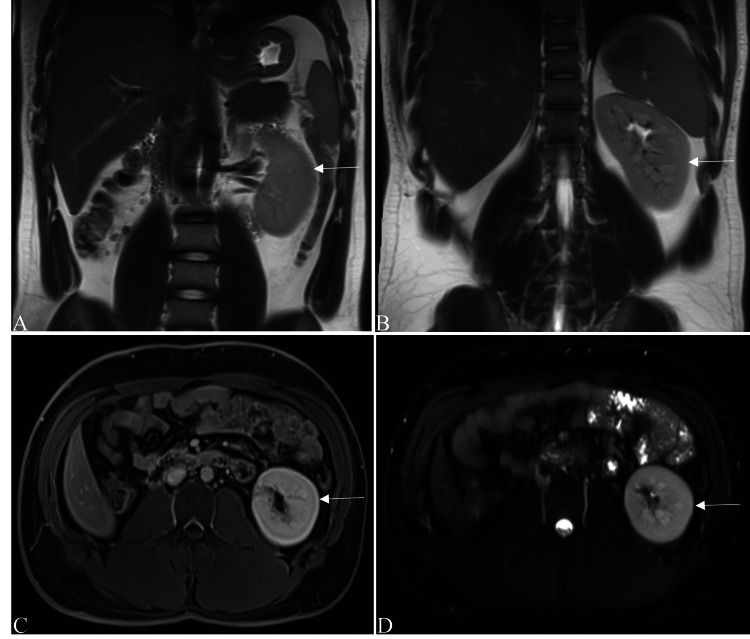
MRI of the abdomen. (A, B) Coronal T2W images, (C) axial post-contrast T1W image, and (D) axial STIR image showed non-visualization of the right kidney and compensatory hypertrophy of the left kidney (white arrows). T1W: T1-weighted; T2W: T2-weighted; STIR: short tau inversion recovery.
